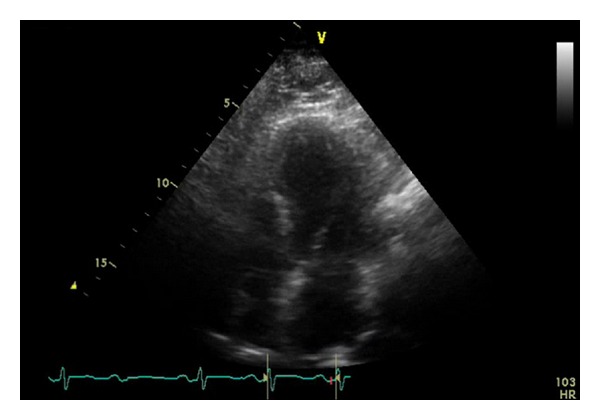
Transthoracic echocardiogram. The patient's transthoracic echocardiogram in diastole showing neurogenic stunned myocardium. Apical four-chamber view showing left ventricle, left atrium, and mitral valve during diastole. Note the uniformity of the endomyocardium resulting in a “bullet shape” of the left ventricle in which all three segments of the ventricle: base, mid, and apex relax equally.
